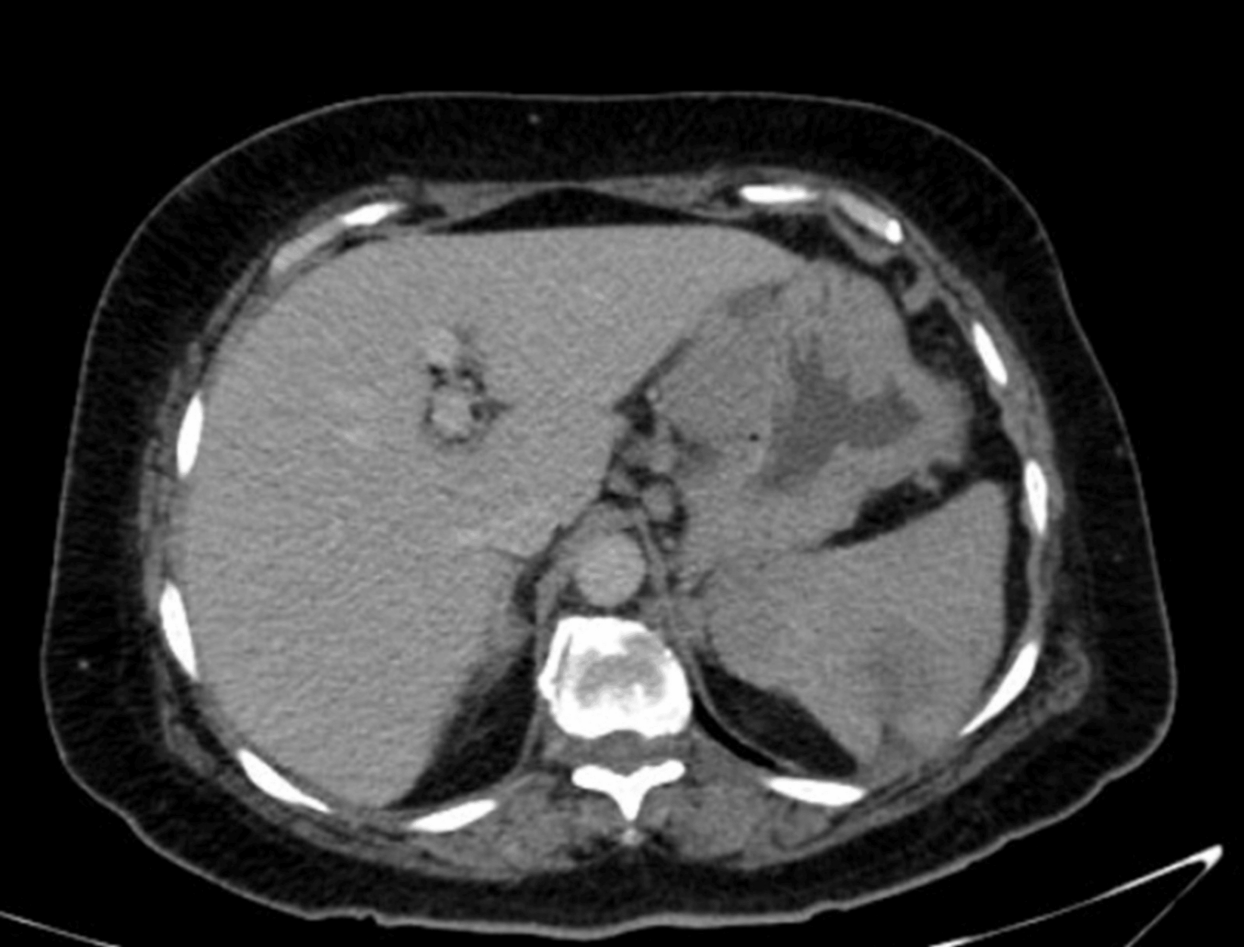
Contrast-enhanced thoracoabdominal computed tomography showing a central splenic infarct and para-aortic lymphadenopathy.
